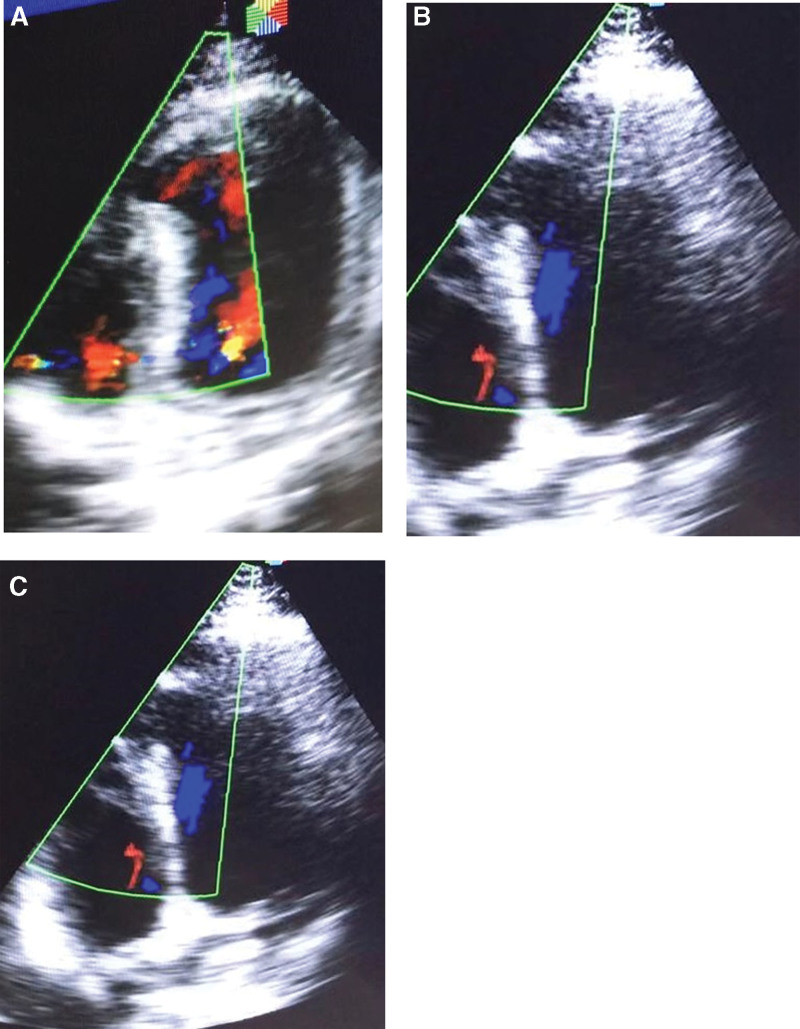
(a, b, c) Echocardiography of a patient with Takotsubo syndrome.
